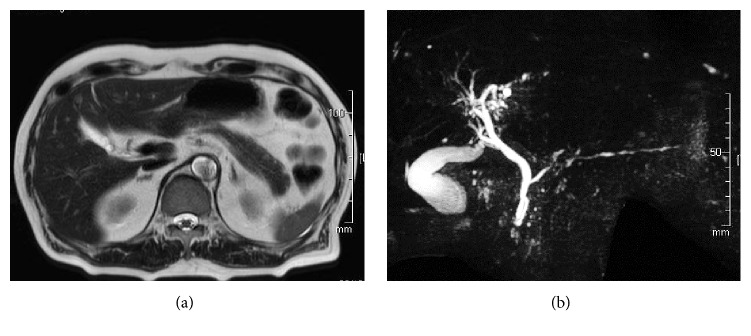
(a) Magnetic resonance image (T2 weighted image) of the abdomen after prednisolone therapy reveals amelioration of the diffuse swelling that had affected the pancreas from body to tail. (b) MRCP after prednisolone therapy revealed amelioration of the narrowing of the main pancreatic duct.
